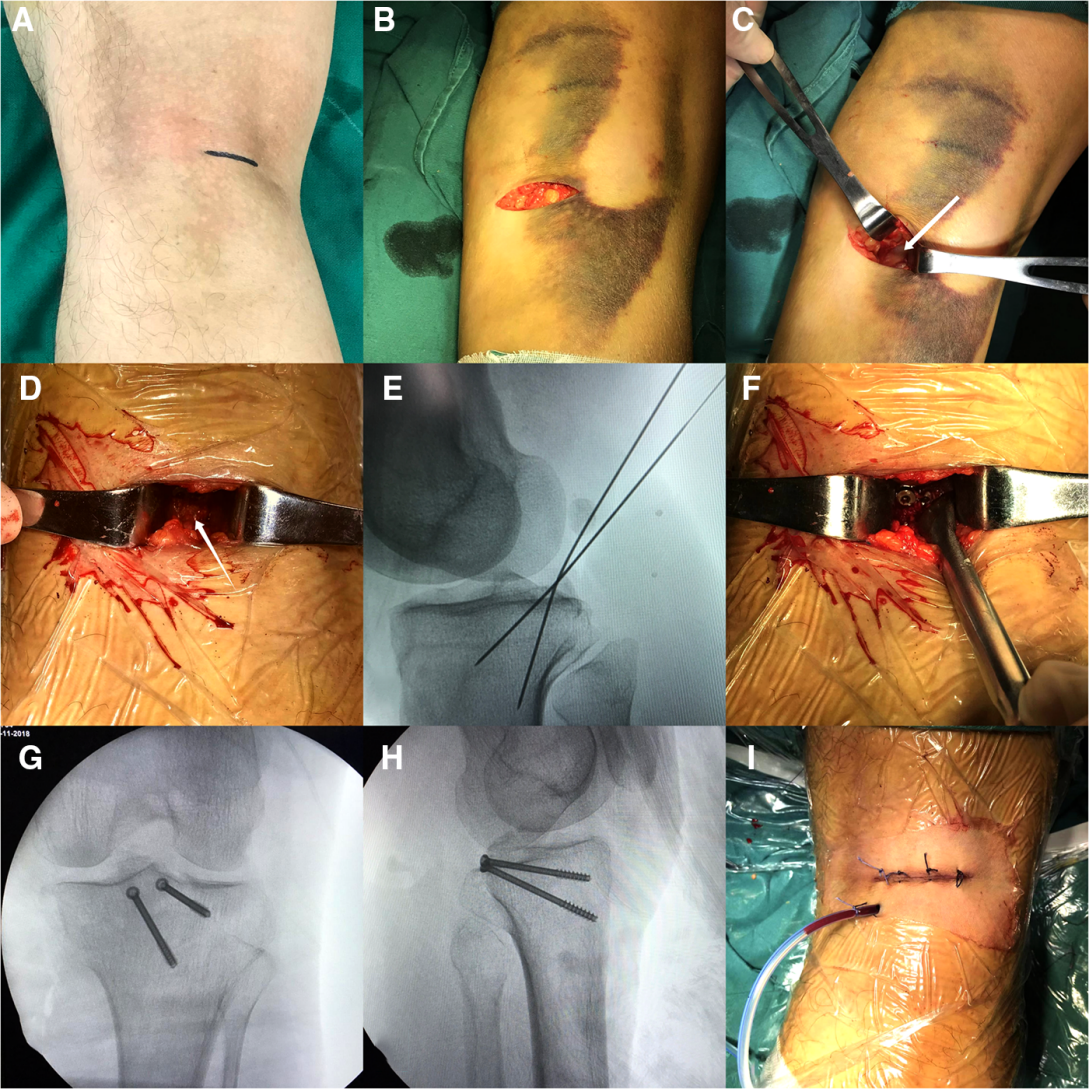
(A–C) A 3–4 cm surgical incision was performed along the preoperative marker, the gastrocnemius muscle's medial head was separated, and the joint capsule was fully exposed by pulling outward. (D–F) The joint capsule was cut open, washed, the fracture block was reduced, and the Kirschner wire was used for temporary fixation. Cannulated lag screws were used for fixation after the fracture was well positioned in fluoroscopic position. (G–I) The intraoperative fluoroscopic anterolateral x-ray film was satisfactory, and the incision was closed layer by layer after rinsing and inserting the drainage tube.
